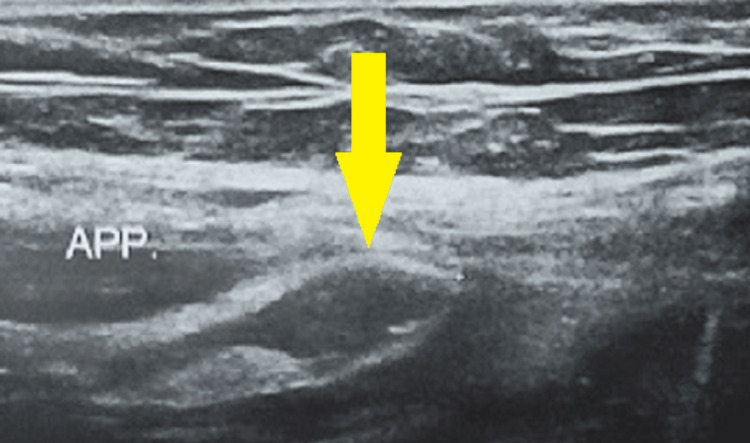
Ultrasonography depicting an inflamed appendix with a 35 mm transverse diameter (arrow) APP.: Appendix
